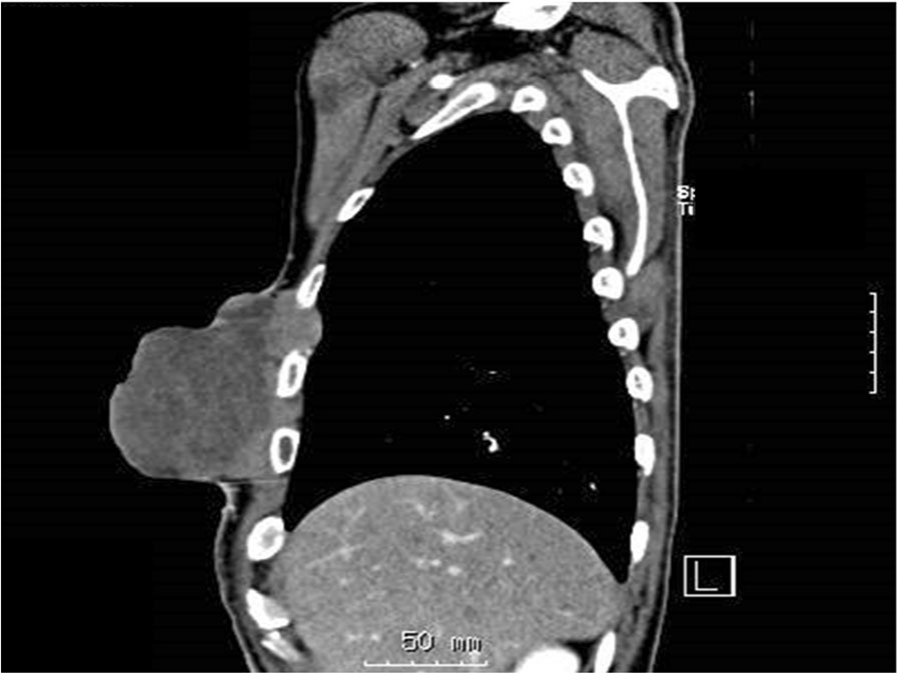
Sagittal computed tomography scan showing the tumor protruding from the chest wall. The tumor also invades the intercostal area
